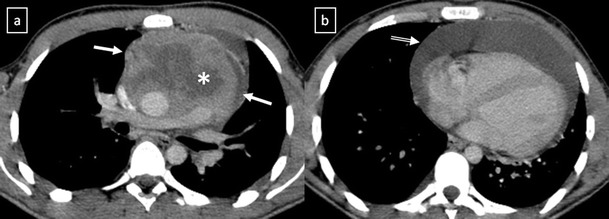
Primary mediastinal diffuse large B-cell lymphoma in a 19-year-old man. Contrast-enhanced CT scan shows a bulky soft tissue mass in the anterior mediastinum (*arrows*in a), which shows heterogeneous CT-attenuation values with cystic changes within (*). Pericardial effusion is also observed (*open arrow* in b)
